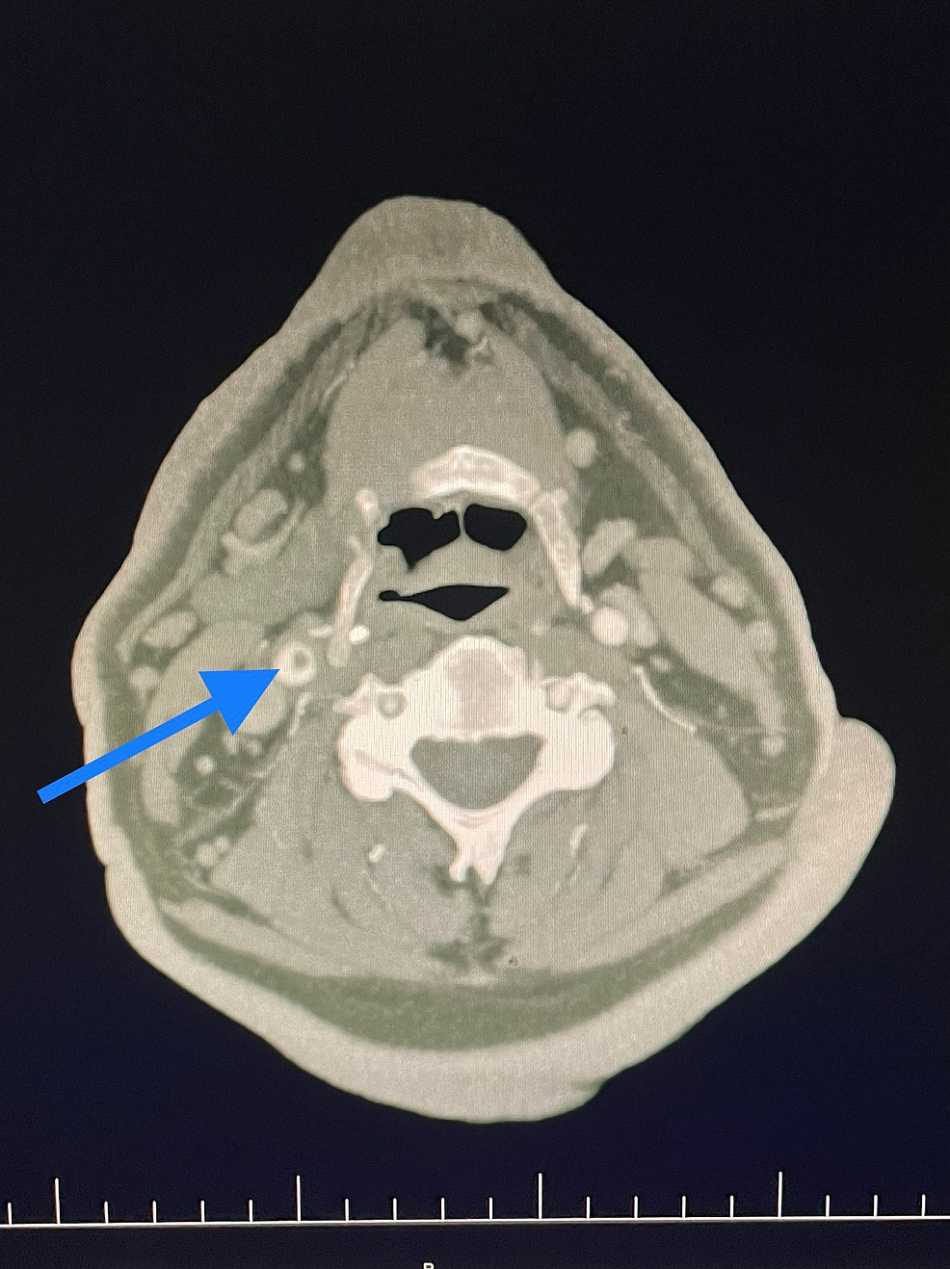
Axial view computed tomography angiogram (CTA) image of the carotid arteries showing a thrombus in the right common carotid artery extending into carotid bifurcation.
